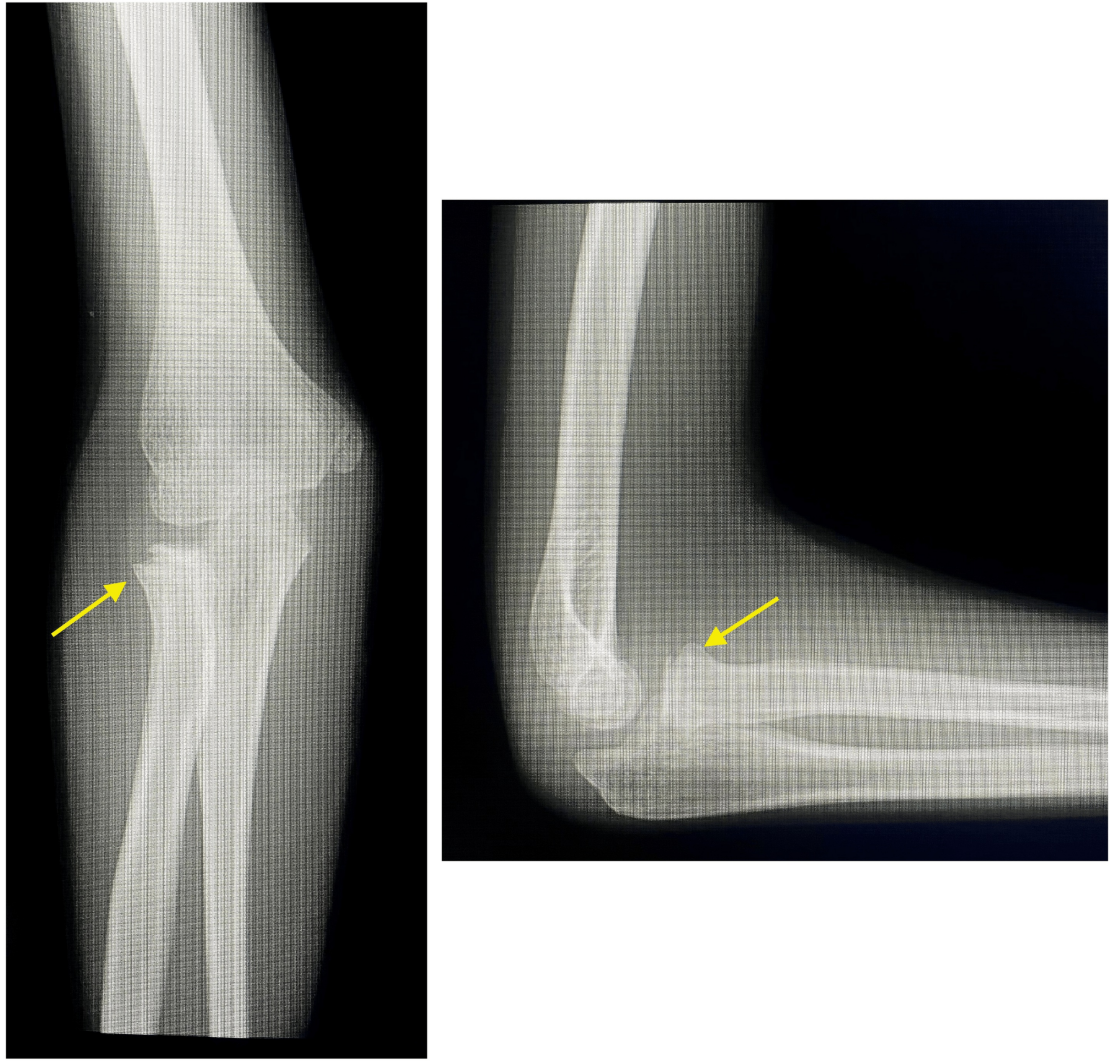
One year after surgery, simple radiographs showed no dislocation and bony fusion.
